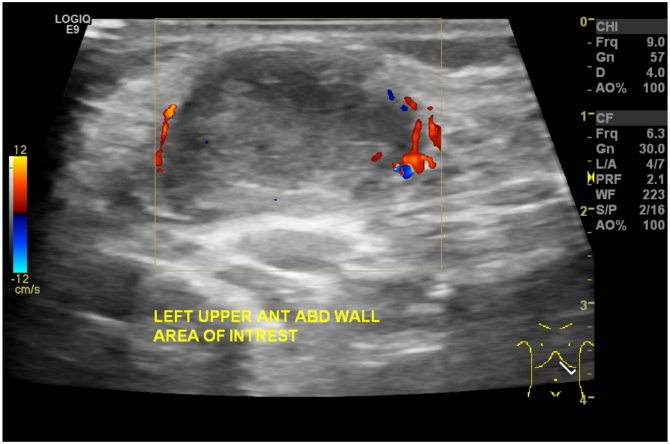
B-mode ultrasound using linear probe showing anterior abdominal wall 3.2 cm rounded well encapsulated heterogeneous predominantly hypoechoic mass with peripheral vascularity is located underneath the skin and displacing the surrounding fat planes.
